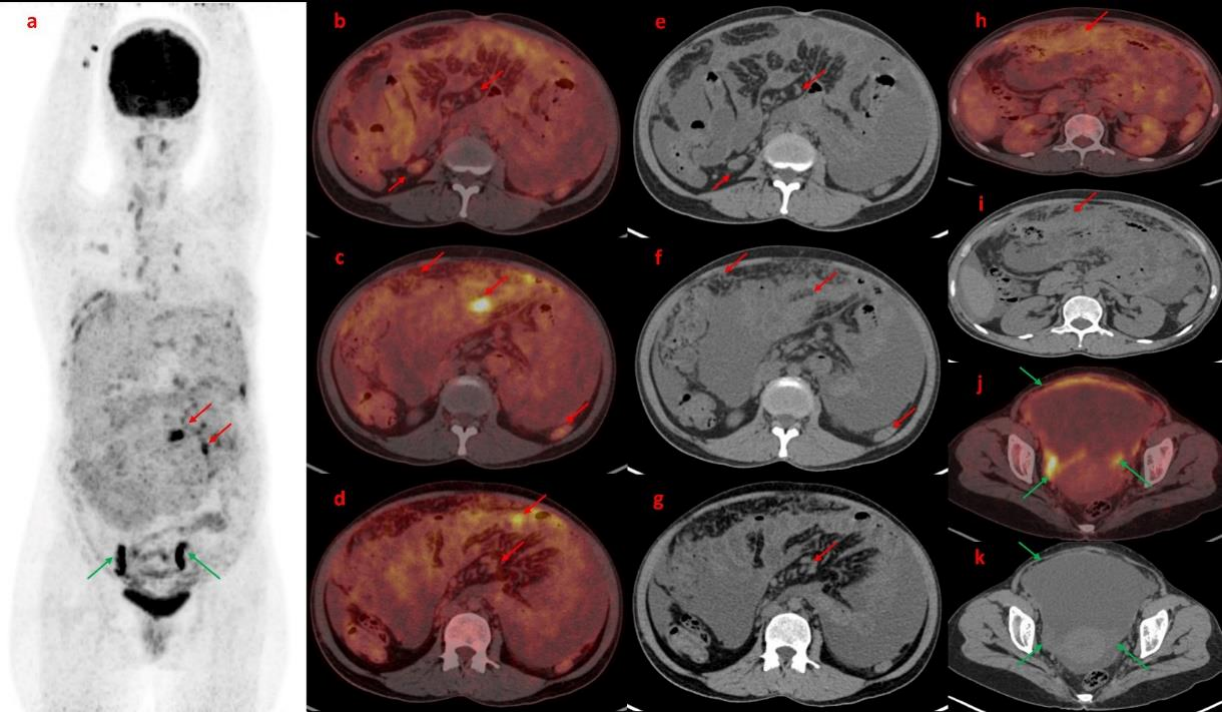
Maximum Intensity Projection (a) image revealed diffuse increased uptake in the peritoneum with few FDG avid nodules and increased FDG uptake in the bilateral adnexal region. (Green arrow). Axial section Fused FDG PET/CT images (b, c, d) and corresponding axial CT images (e, f, g) revealed omental thickening, omental nodules, few peritoneal deposits, and mesenteric thickening with nodularity. Ascites can also be noted in all these sections. Further Fused Axial PET/Ct images (h, j) and axial CT images (I, k) revealed omental caking and peritoneal thickening with increased FDG uptake (red arrow) and ascites. A fused axial PET/CT image (j) and corresponding axial CT image (k) also revealed an FDG avid bilateral adnexal lesion with pelvic ascites
